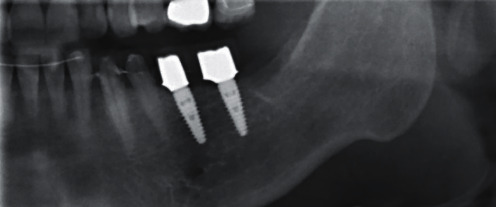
X-ray check during LTT fitting.
